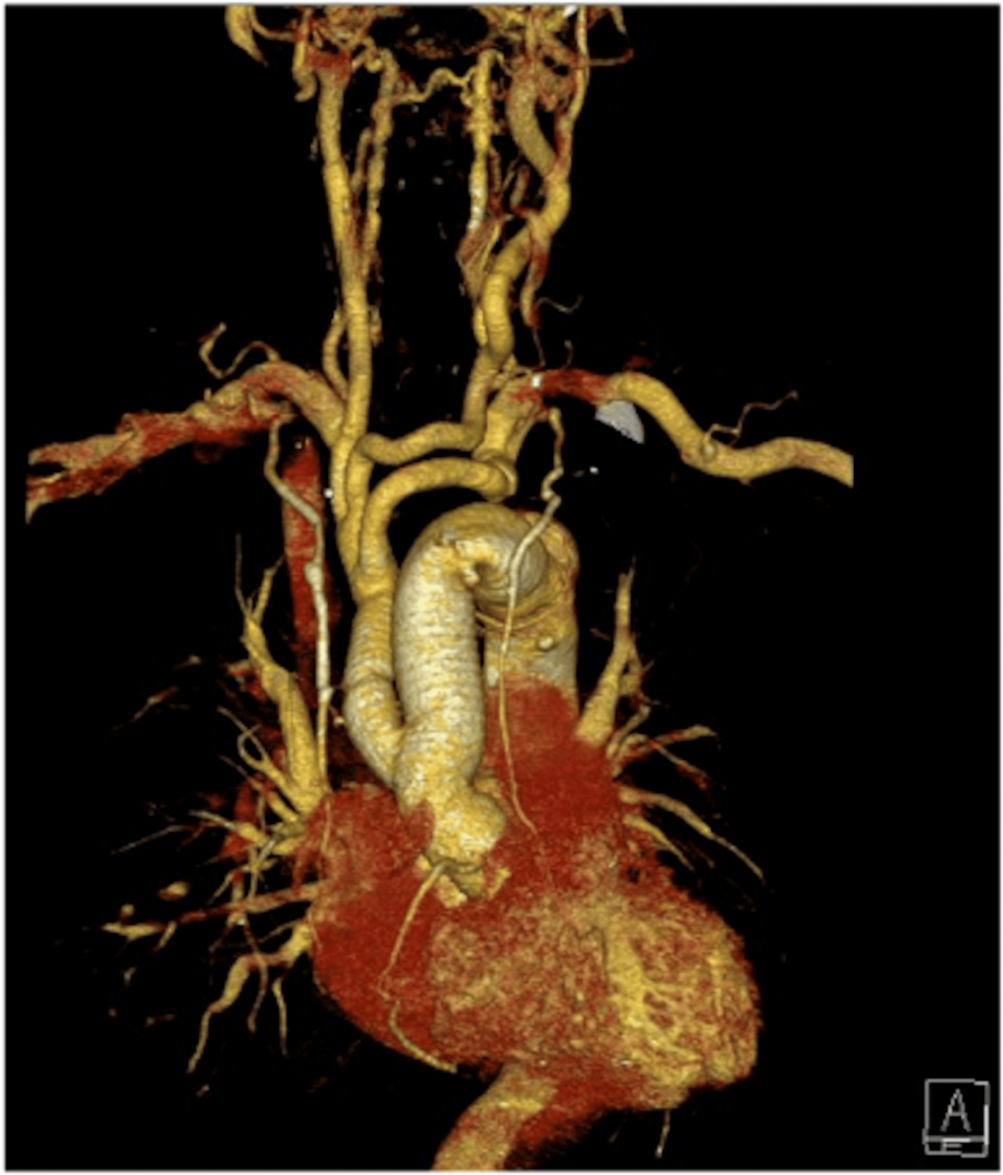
Postoperative CT angiography showing a complete repair of the aortic dissection using the branch-first technique
